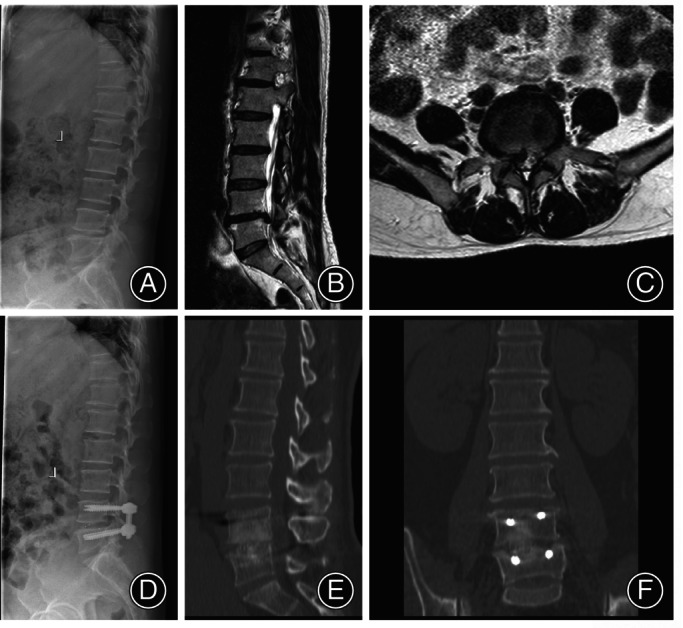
51‐year‐old female, chronic low back pain with right lower extremity radiating pain treated by MIS‐TLIF. (A–C) Lateral radiograph, the sagittal and axial MRI images demonstrate central canal and right lateral recess stenosis with the sequestered disc at the L4/5 level. (D) Six months after surgery, the X‐ray film shows excellent restoration of disc height and pedicle screw position. (E, F) Three‐year postoperative CT image showing interbody fusion has been achieved.
